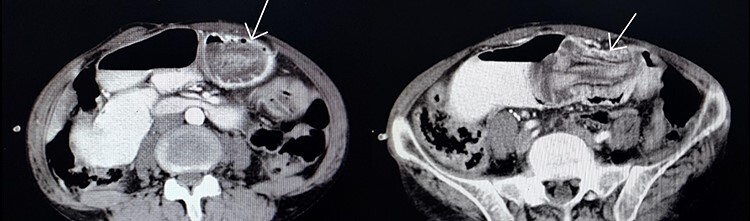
Jejunogastric intussusception showed (arrow) in abdominopelvic CT scan with Iv/oral contrast.
